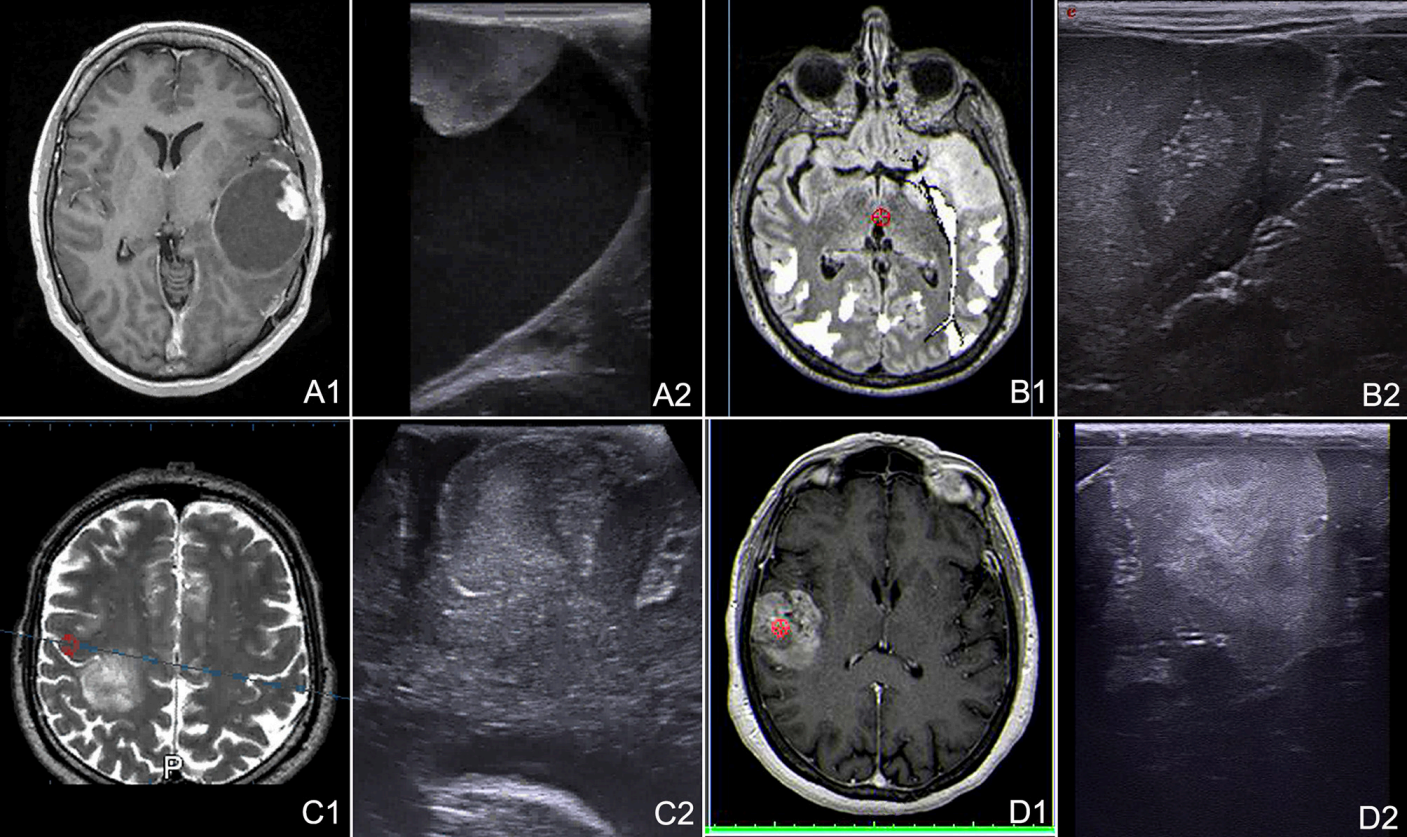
B-mode representation of different glioma grades. (A) Left temporal pilocytic astrocytoma. (B) Left temporal diffuse astrocytoma. (C) Right parietal anaplastic astrocytoma. (D) Right temporo-parietal glioblastoma. (1) pre-operative volumetric MRI. (2) Intra-operative trans-dural US scan. Note the different lesion appearances and in particular the different degree of margins definition.
